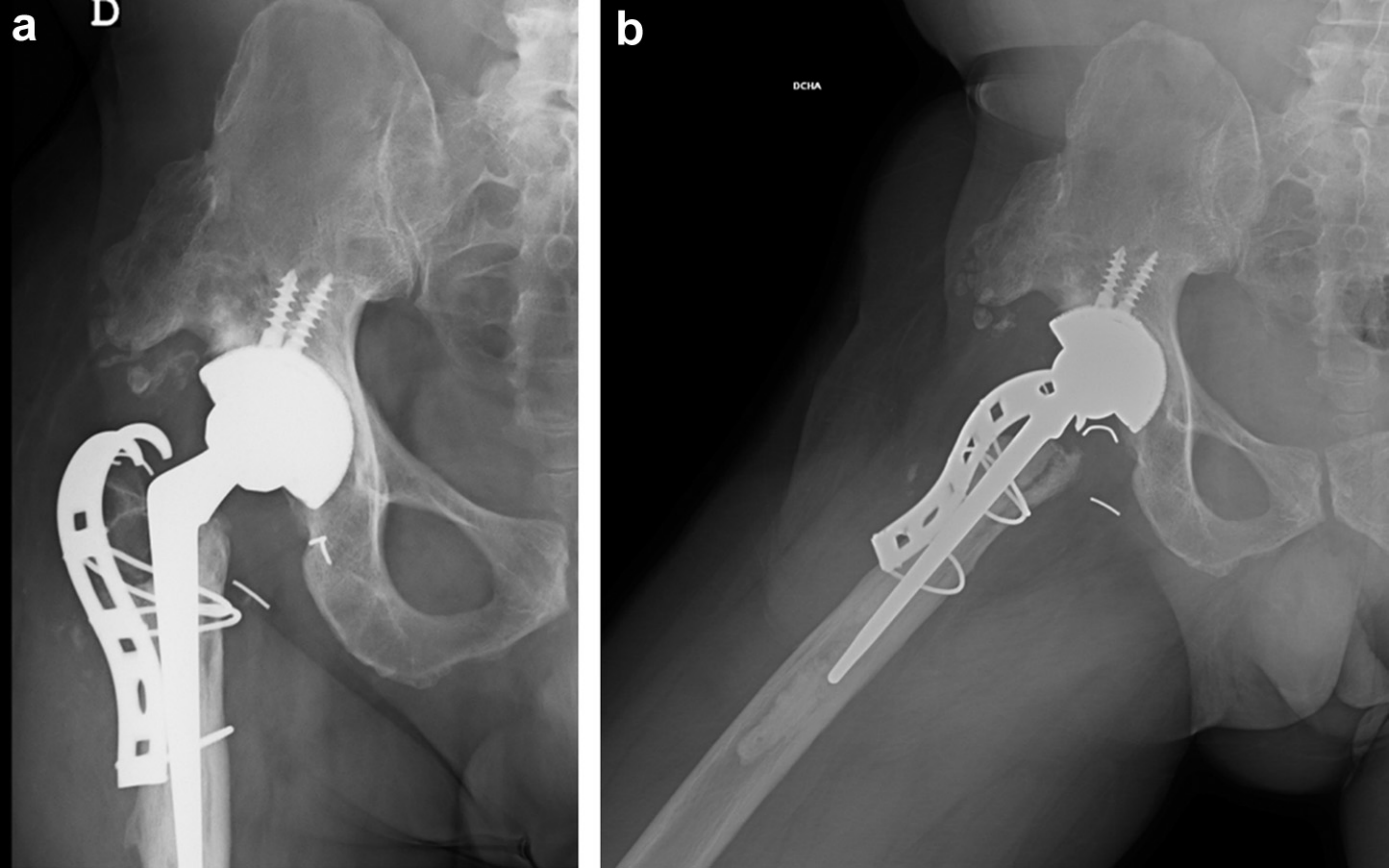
Anteroposterior (a) and frog-leg lateral (b) radiographies of the pelvis demonstrating good concentric reduction of the dislocation.
